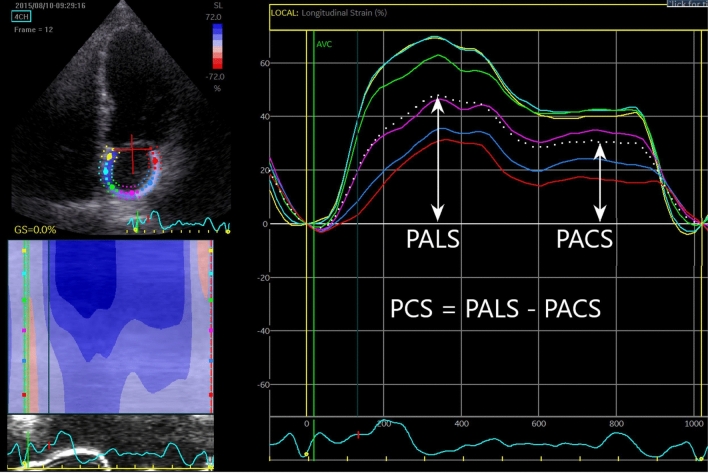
Example of left atrial speckle tracking echocardiography. Example of left atrial speckle tracking from the apical 4-chamber view. Coloured lines represent each segment, and the white dotted line represents the global value
